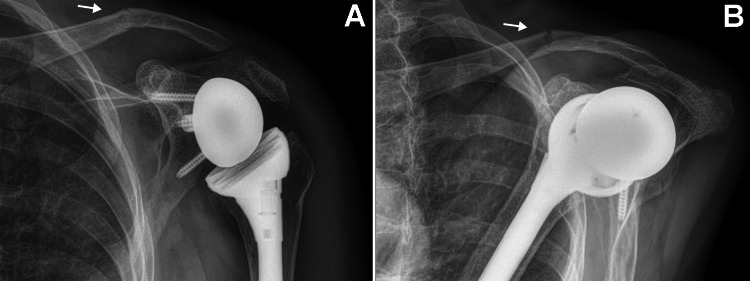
Radiographs taken six months after RTSA. Radiographs of the left shoulder taken six months after the RTSA showing a mildly angulated fracture of the clavicle (arrows) in addition to the angulated fracture of the acromion. The clavicle fracture is in the vicinity of the prior (healed) clavicle fracture, as seen in Figure 1. (A) Anterior-posterior view and (B) scapula Y view. RTSA: Reverse total shoulder arthroplasty
